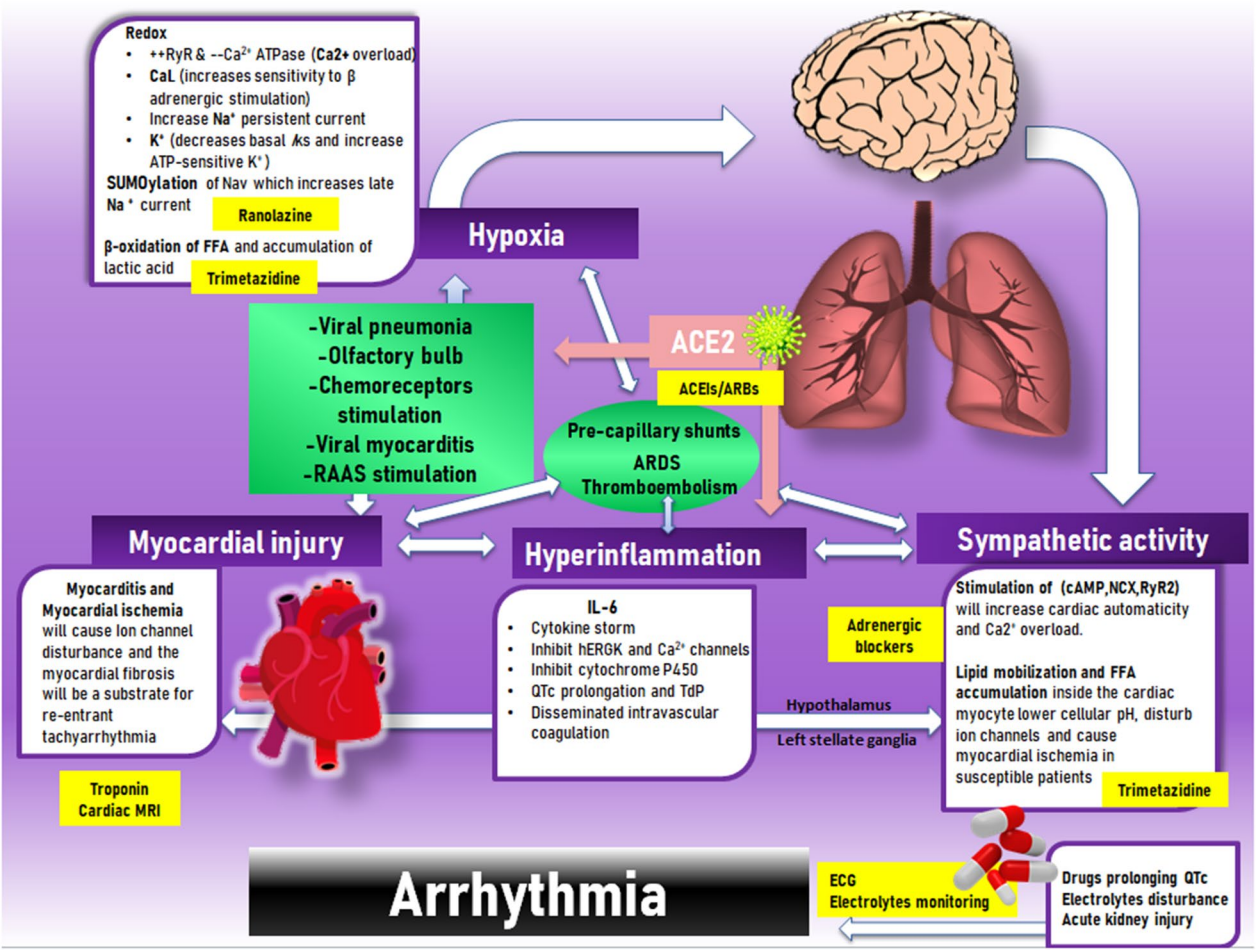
Mechanism of arrhythmia in COVID-19 infection and the proposed management protocol. ACEIs: angiotensin converting enzyme inhibitors; ARBS: angiotensin receptor blockers; ARDS: adult respiratory distress syndrome; ATP: adenosine tri-phosphate; FFA: Free fatty acid; hERG: Human ether-a-go-go-related gene; IL-6: Interleukin-6; MRI: magnetic resonance imaging; Nav: voltage gated Na^+^ channels. RAAS: renin angiotensin aldosterone system; RyR: Ryanoide receptors; Tdp: Torsade de points
